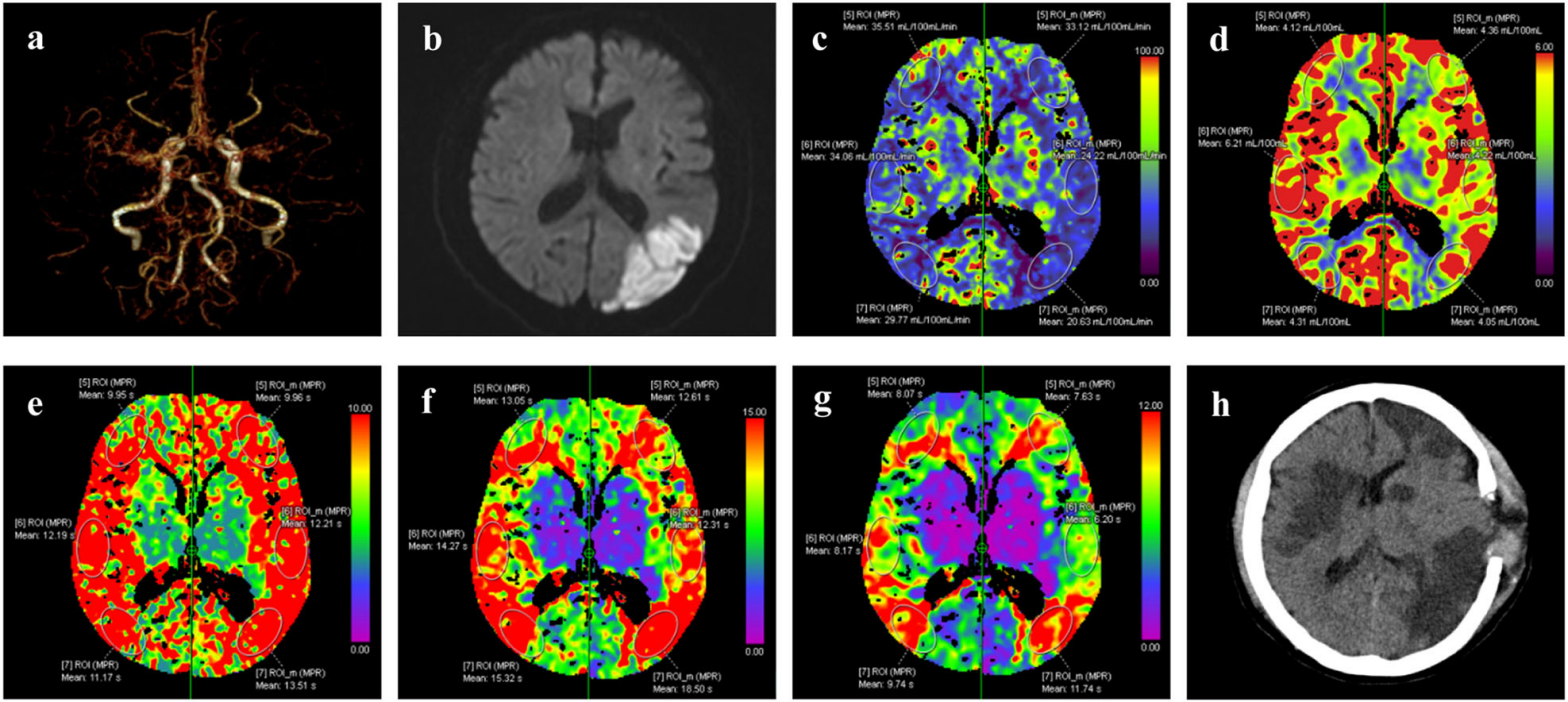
A 50-year-old male MMD patient with post-CI. Preoperative CTA showed MMD (a). The diffusion-weighted imaging performed two weeks before surgery demonstrated an acute cerebral infarction in the left parietal-occipital lobe (b). Preoperative CTP showed a significant decrease in CBF (c), a mild decrease in CBV (d), and a significant increase in MTT (e), TTD (f), and Tmax (g) in both hemispheres. Right hemiplegia appeared on the second day after surgery, followed by coma. Postoperative CT showed multiple newly developed cerebral infarctions in both hemispheres (h)
